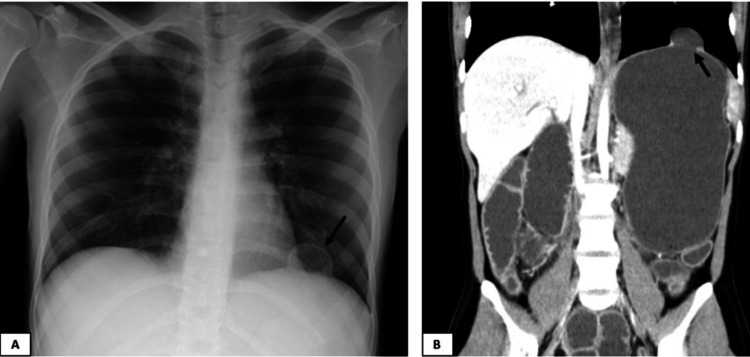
Admission chest X-ray and abdominal CT scan (A) Admission standard chest X-ray showing a small diaphragmatic hernia (arrow); (B) CT scan showing enlargement of the stomach, the bowel, and a small left diaphragmatic hernia (arrow).
